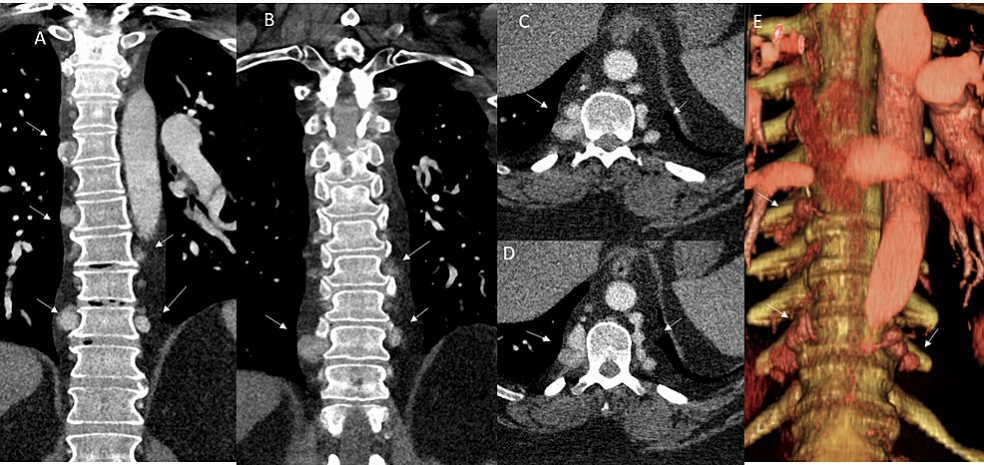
Intercostal artery aneurysmosis on CT angiogram Multiple coronal (A, B) and axial (C, D) CT angiogram images reveal multiple variable-sized intercostal artery aneurysms in the bilateral paravertebral region from the fifth to the tenth (T5-T10) vertebral levels, measuring 0.4-1.7 cm in diameter (arrows). The intercostal origin of these aneurysms was confirmed on the source angiogram and colored volume-rendered image (E).
